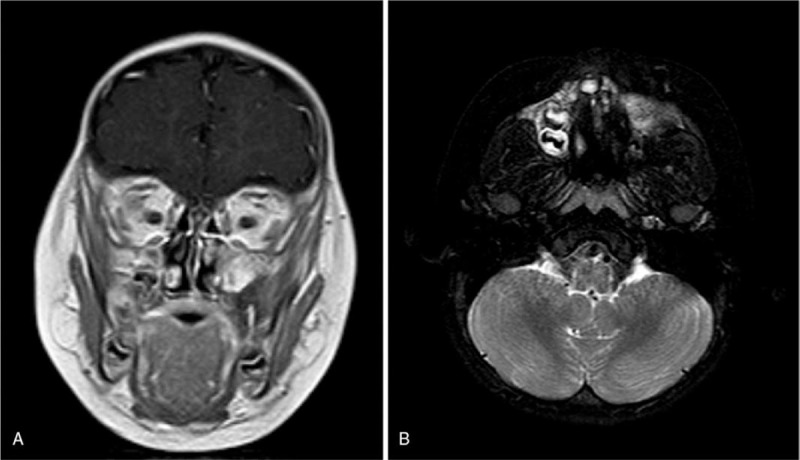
After 9 months of metformin treatment, the nasopharynx MRI (2020.1.8) showed that the size of the tumor was unchanged and was the same as seen on April 9, 2019.
